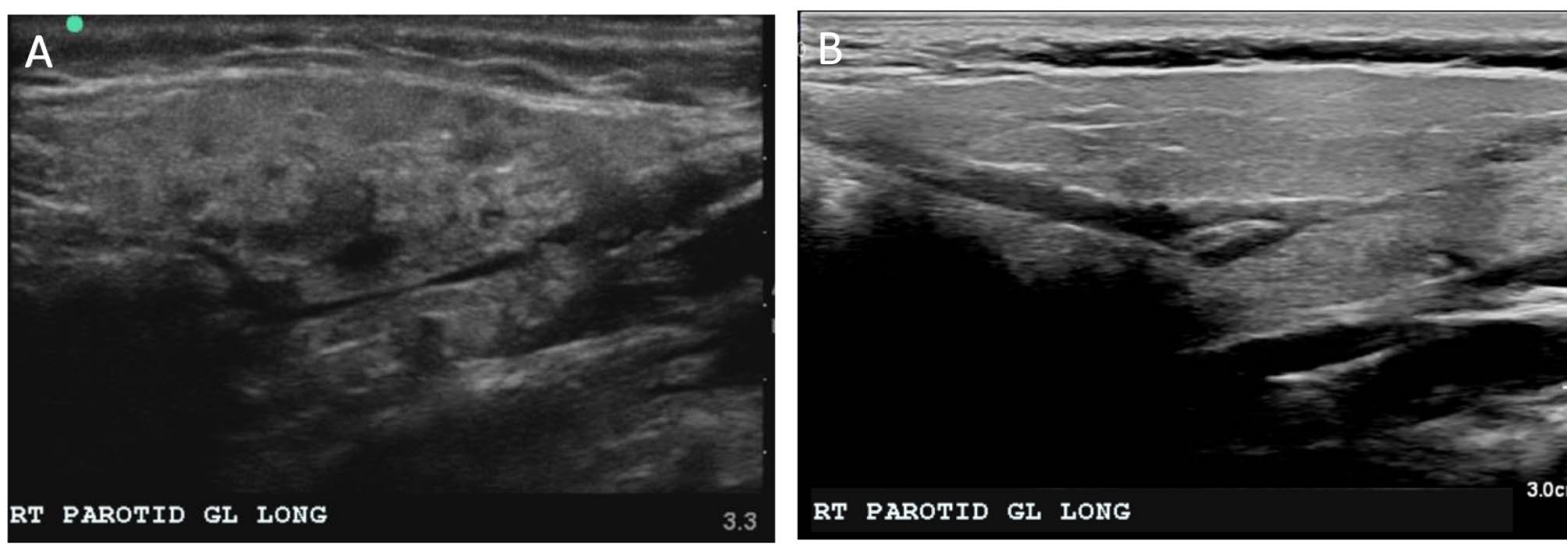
Right parotid ultrasound at baseline before sialendoscopy with SGUS score of 16 (A) in comparison with a healthy individual of same age (B)
